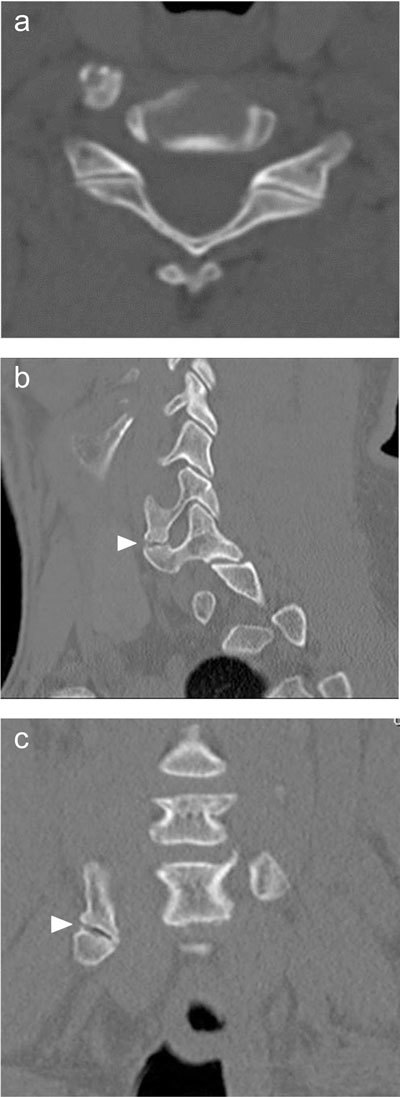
(a–c) Cervical CT with axial, sagittal and coronal reformations more clearly shows a right-sided elongation of the anterior tubercle of the transverse process of C6 and C7 and an accessory articulation between these anterior transverse processes (arrowhead).
